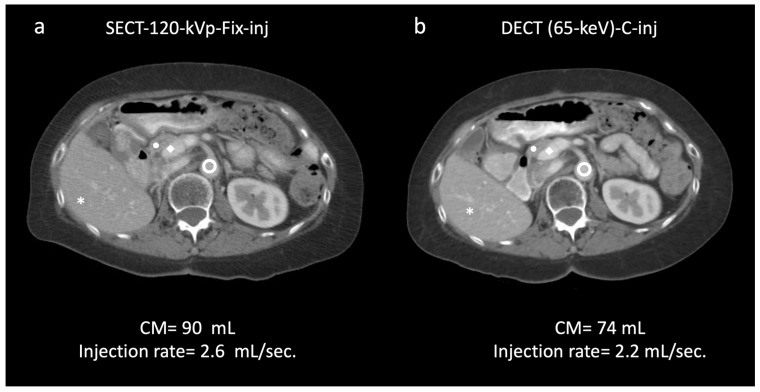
Clinical example of a 58-year-old female (68 kg). A 120 kVp single-energy CT (SECT) with fixed injection (90 mL) demonstrated the following attenuation (HU)/contrast-to-noise ratio (CNR) values: * liver, 114.9/22.5; ● pancreas, 95.4/19.6;
